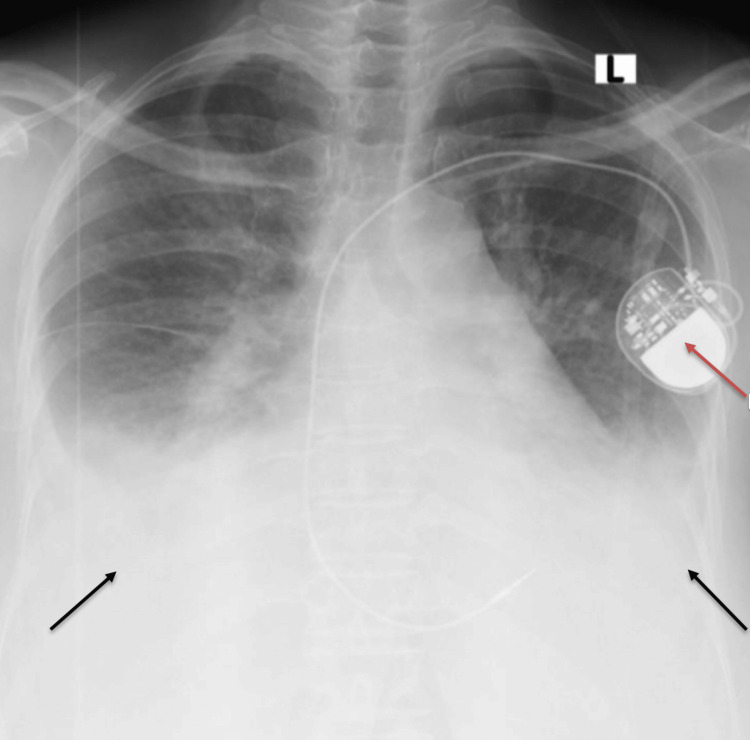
Frontal chest radiograph of the patient The image shows a single-chamber pace maker in situ (red arrow). Bilateral lower zone opacities (black arrows) are noted with obliteration of the costophrenic angles and diaphragmatic borders, consistent with bilateral pleural effusions.
